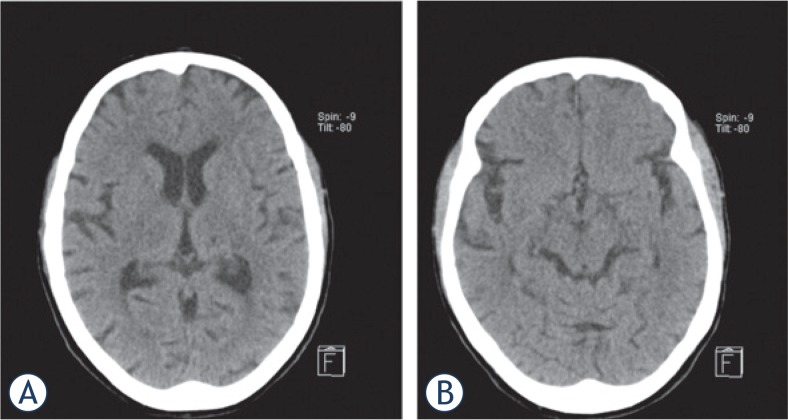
The non-contrast head CT scan performed on the day of admission was normal, in particular without early signs of ischemia at the level of both thalami (A) the mesencephalon (B).
